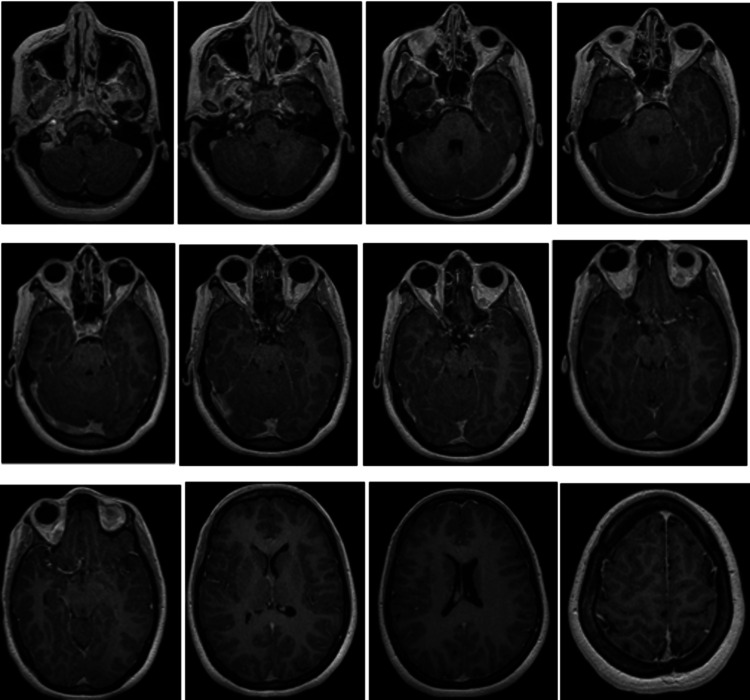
Post-contrast sagittal T1-weighted MRI showing intramedullary enhancement Post-contrast sagittal T1-weighted image demonstrating patchy intramedullary enhancement supporting inflammatory etiology (arrow). MRI: Magnetic resonance imaging
